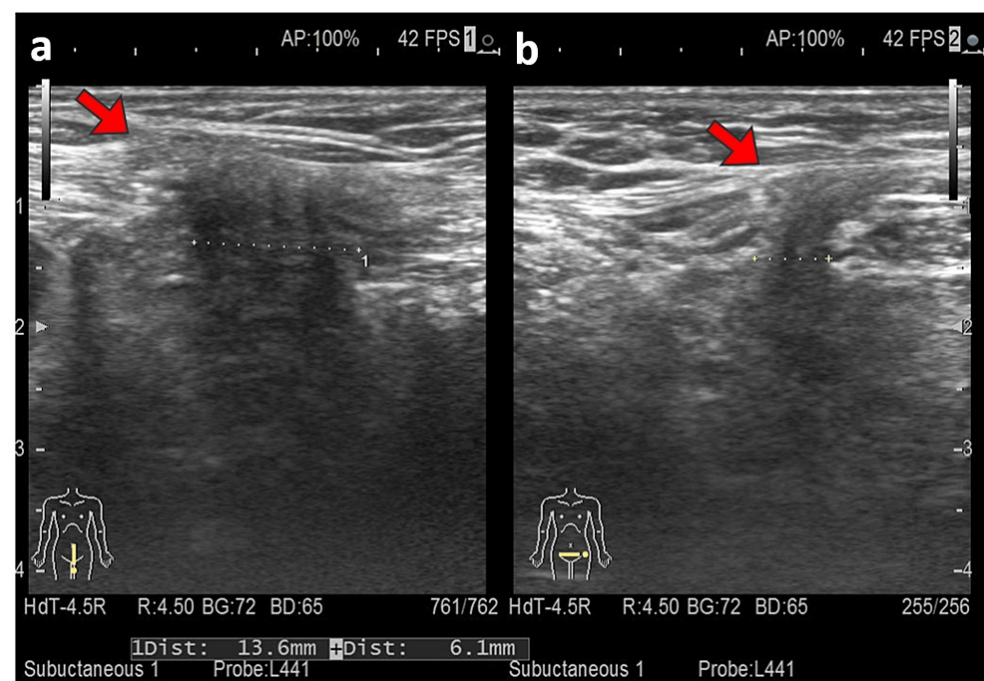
Ultrasonography revealing the presence of a hernia. The abdominal incisional hernia was observed. The hernia portal was about 1.3 cm × 6.1 mm.
